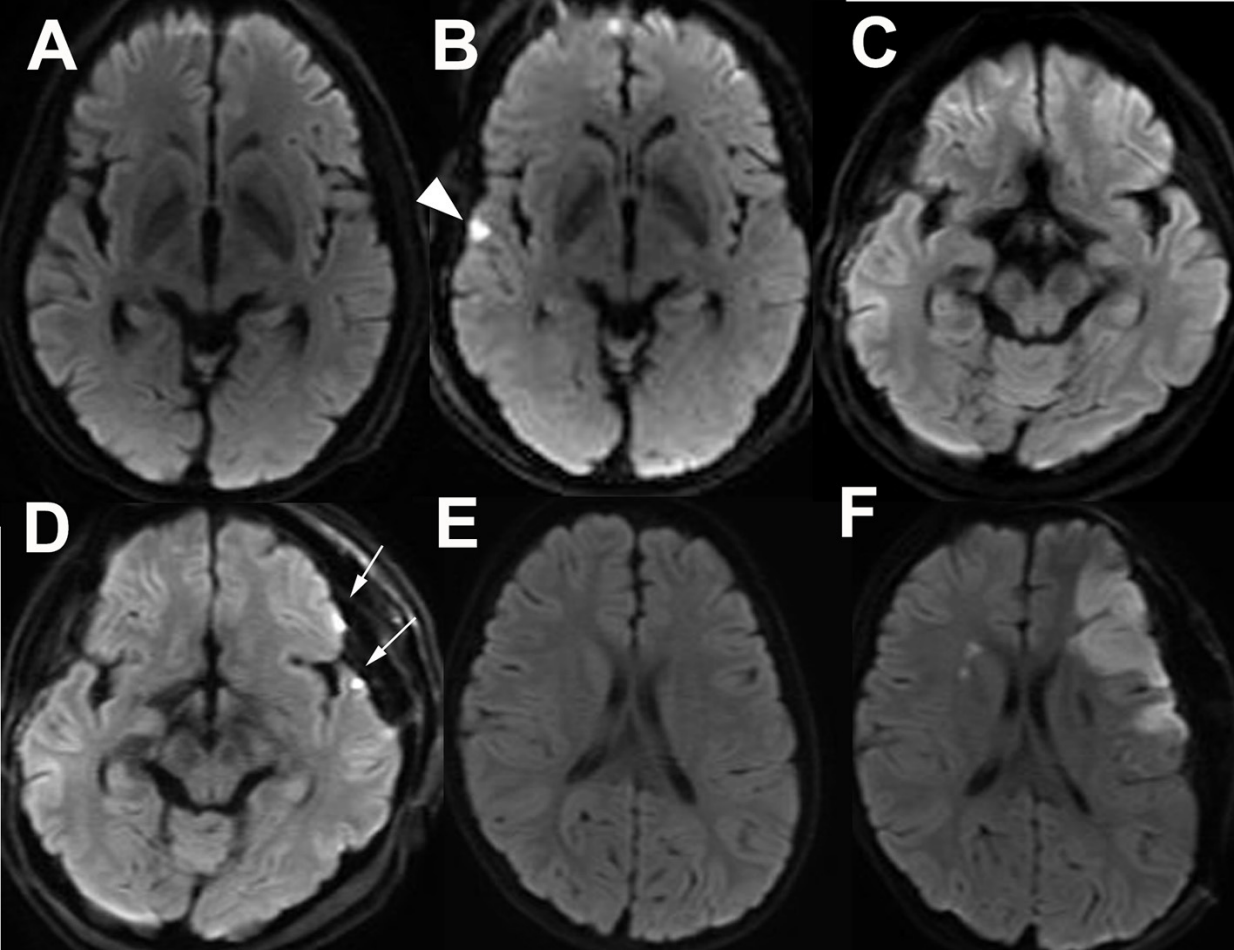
Asymptomatic small infarct lesion at the frontal and or temporal lobes adjacent to the Sylvian fissure detected by postoperative diffusion-weighted imaging (DWI) is defined as an anastomotic site infarction (ASI). Preoperative (A) and postoperative (B) DWI of a patient with temporal ASI (arrow head). Preoperative (C) and postoperative (D) DWI of a patient with frontal and temporal ASIs (arrows). Preoperative (E) and postoperative (F) DWI of a patient with major postoperative infarction at the affected side is distinguished from ASI.
